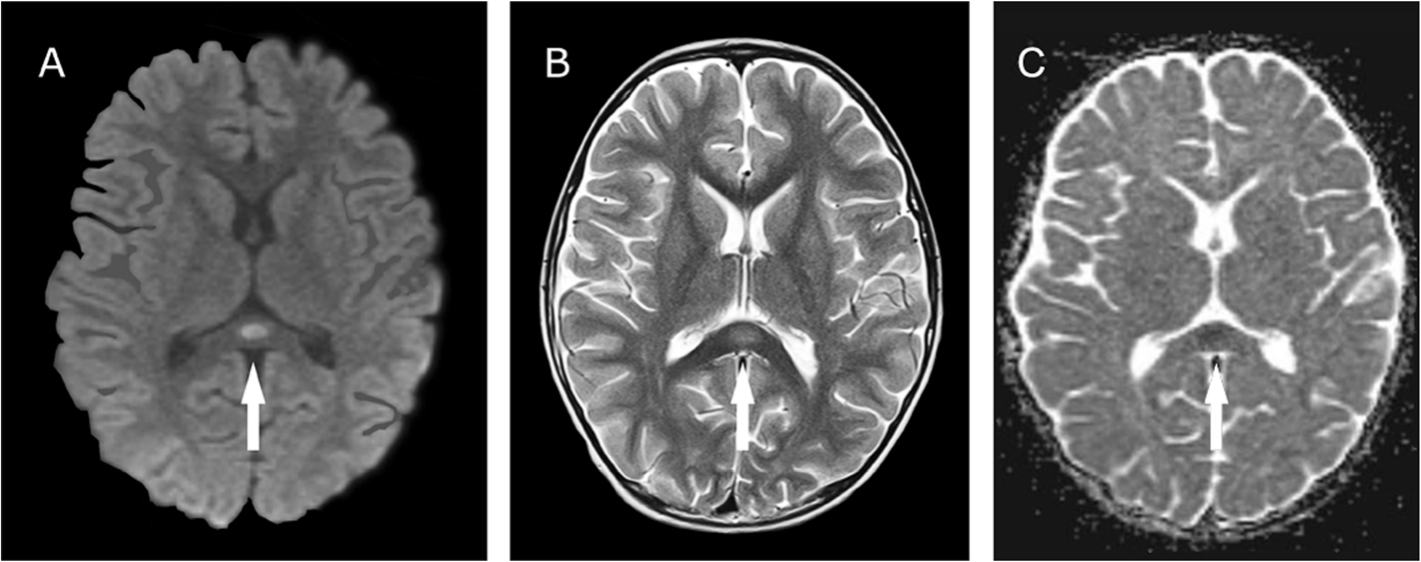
MRI of the brain performed on day 6 of disease a Diffusion-weighted image shows a marked hyperintensity in the SCC. b Corresponding T2 image. c Corresponding diffusion restriction on Apparent Diffusion Coefficient (ADC) mapping
